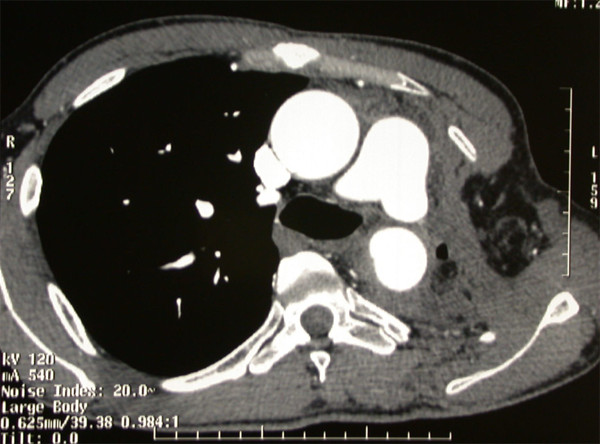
Axial contrast enhanced CT shows the remodelling osteomuscular wall of the thoracic cage with a collapse of left pleural space.
